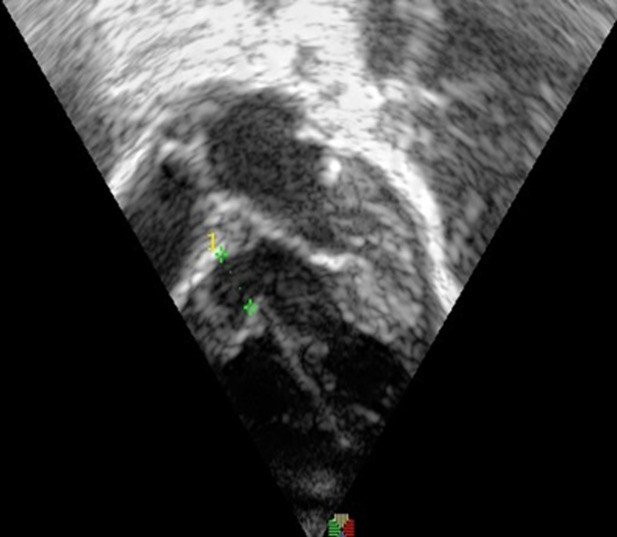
pre-procedure echocardiogram
